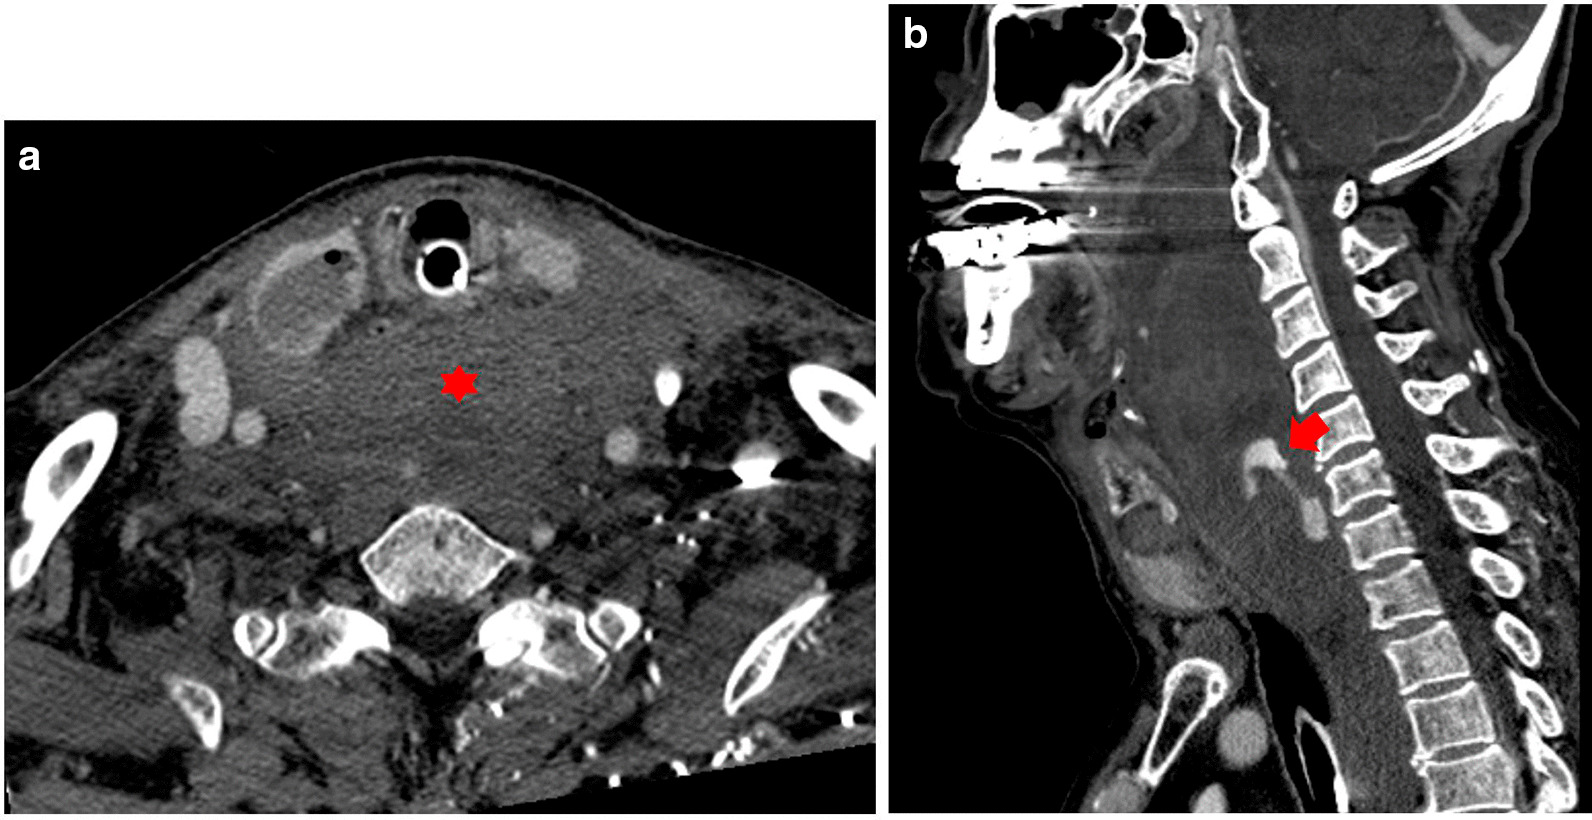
a An axial neck CT take after thyroid FNA underwent on emergency department reveals a large hematoma (red asterisk) in the anterior neck space with anterior tracheal deviation and nodule in the right thyroid lobe with intra- and extra nodule air-bubbles caused by the fine needle aspiration. b Sagittal CT scan shows extravasation of contrast media suggesting active bleeding (red arrow) within the hematoma
